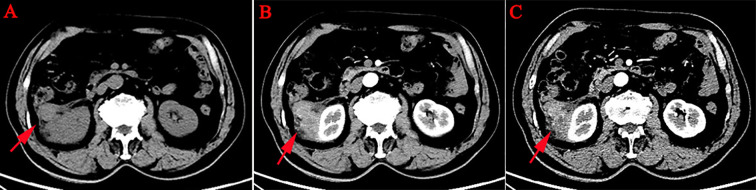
Abdominal CT. This image showed flocculent, nodular, and cord-like mixed-density lesions in the right perirenal space. The largest nodule measured approximately 3.3 cm × 2.7 cm with ill-defined borders, causing slight compression of the right kidney, and the solid components of the lesions showed enhancement. Additionally, the right lateral conical fascia and prerenal fascia were thickened, with obvious enhancement of the nodular shadows within them [(A–C), red arrow].
